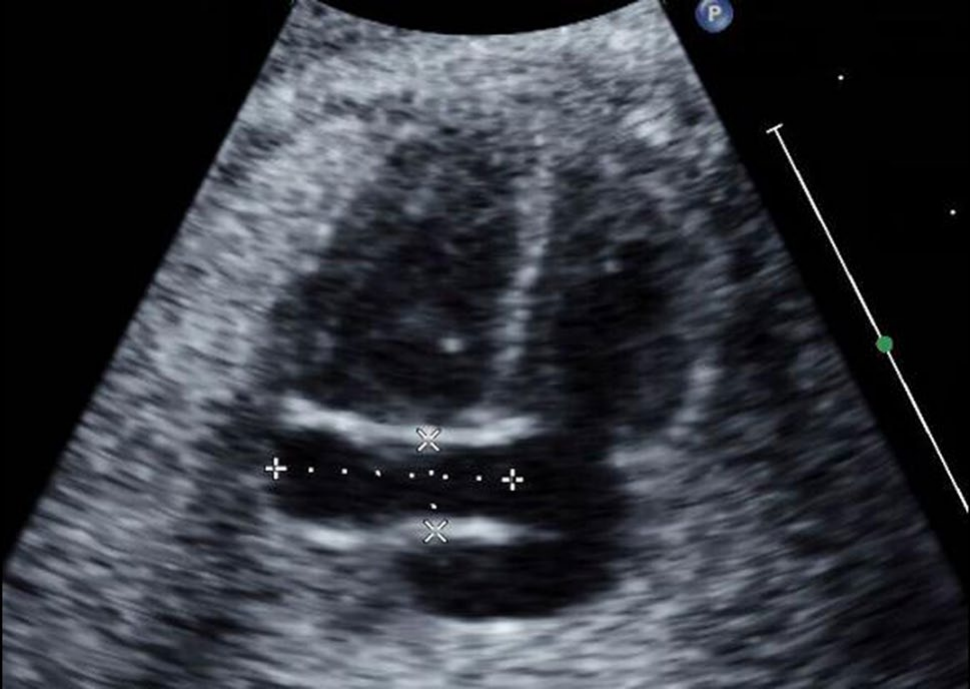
A standard fetal apical four-chamber view. Dilatation of the coronary sinus with PLSVC can be visualized
